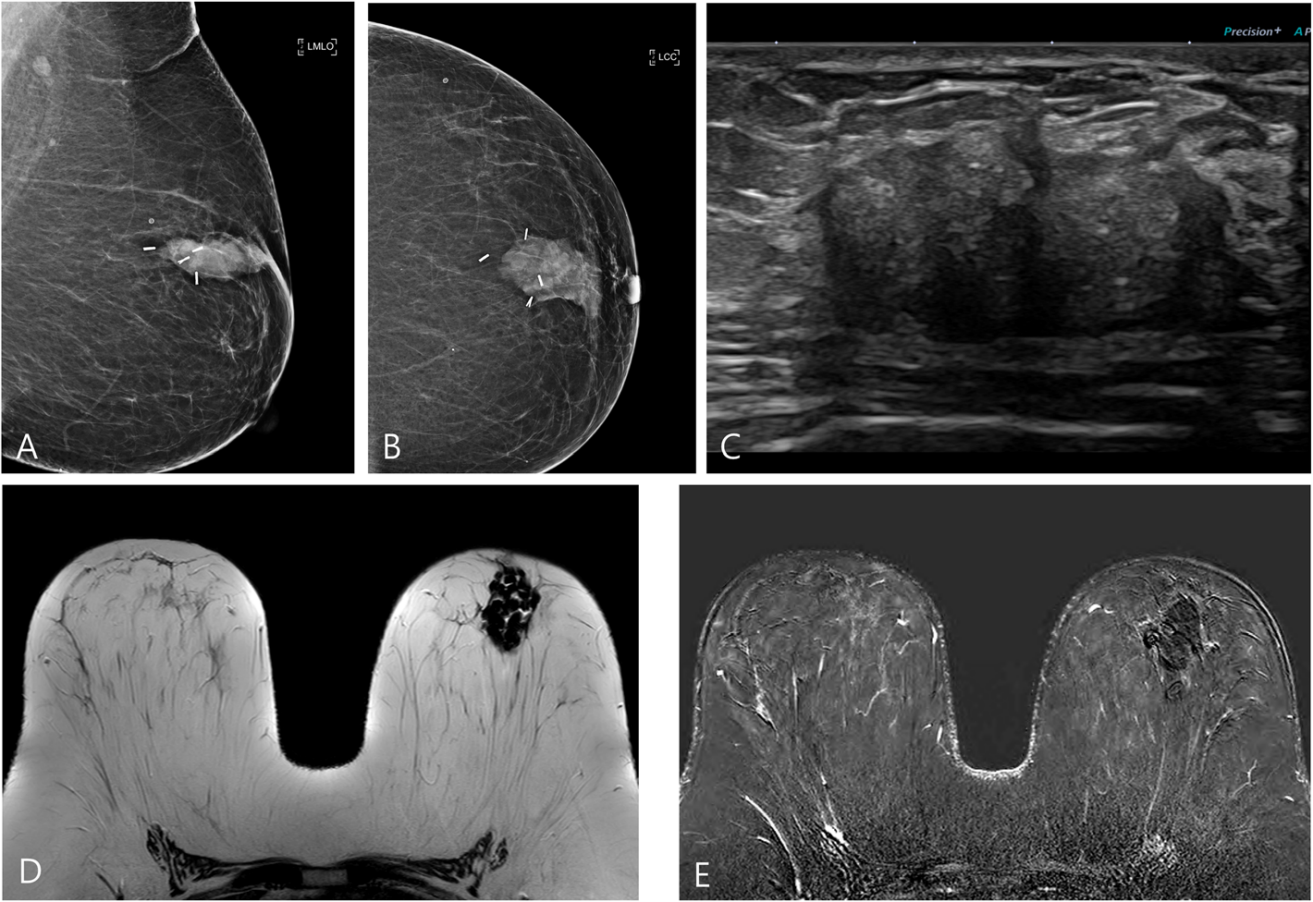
Six-month follow-up studies of a 42-year-old woman who underwent breast-conserving surgery with ADM. Mammography showed ADM as a mass with a well circumscribed margin on the left mediolateral oblique view (a) and left cranio-caudal view (b). The diced ADM was observed to the same echogenicity as fibroglandular parenchyma on B-mode ultrasonography (c). The ADM showed low signal intensity on T2-weighted imaging (d) and no enhancement on contrast-enhanced magnetic resonance imaging (e). ADM = acellular dermal matrix
